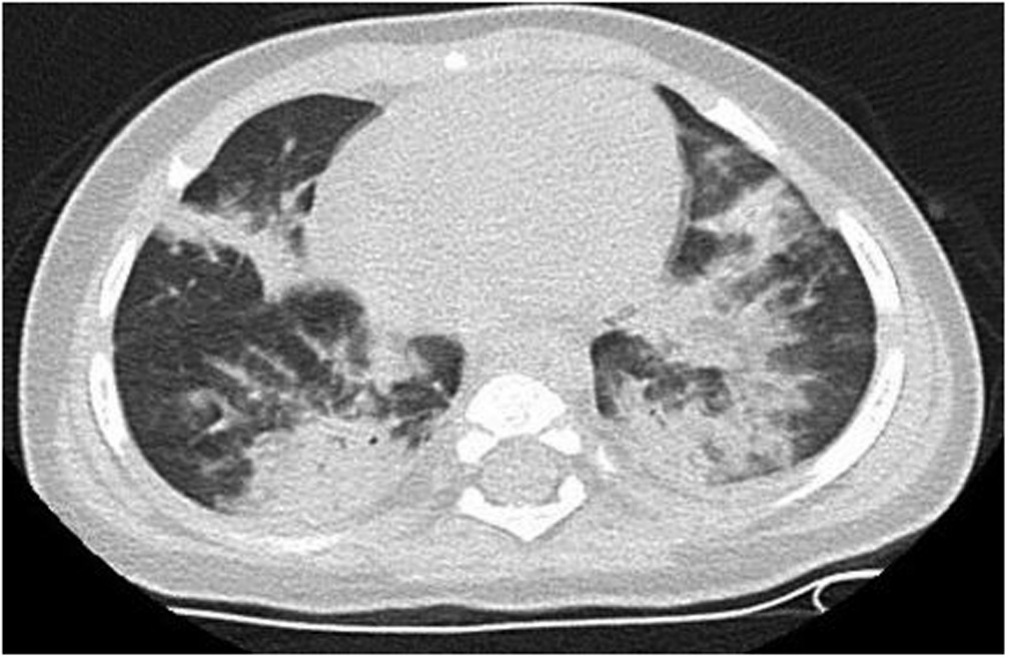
High-resolution computed tomography (HRCT) of an 11-month-old boy with epilepsy, hydrocephalus, cognitive/motor delay, sepsis, and COVID-19 pneumonia: Bilateral ground-glass opacities and areas of complete/incomplete consolidation containing sporadic air bronchograms in the basal segments and perihilar region that are more pronounced in the middle and lower zones of the lung parenchyma. COVID-19: coronavirus disease 2019.
